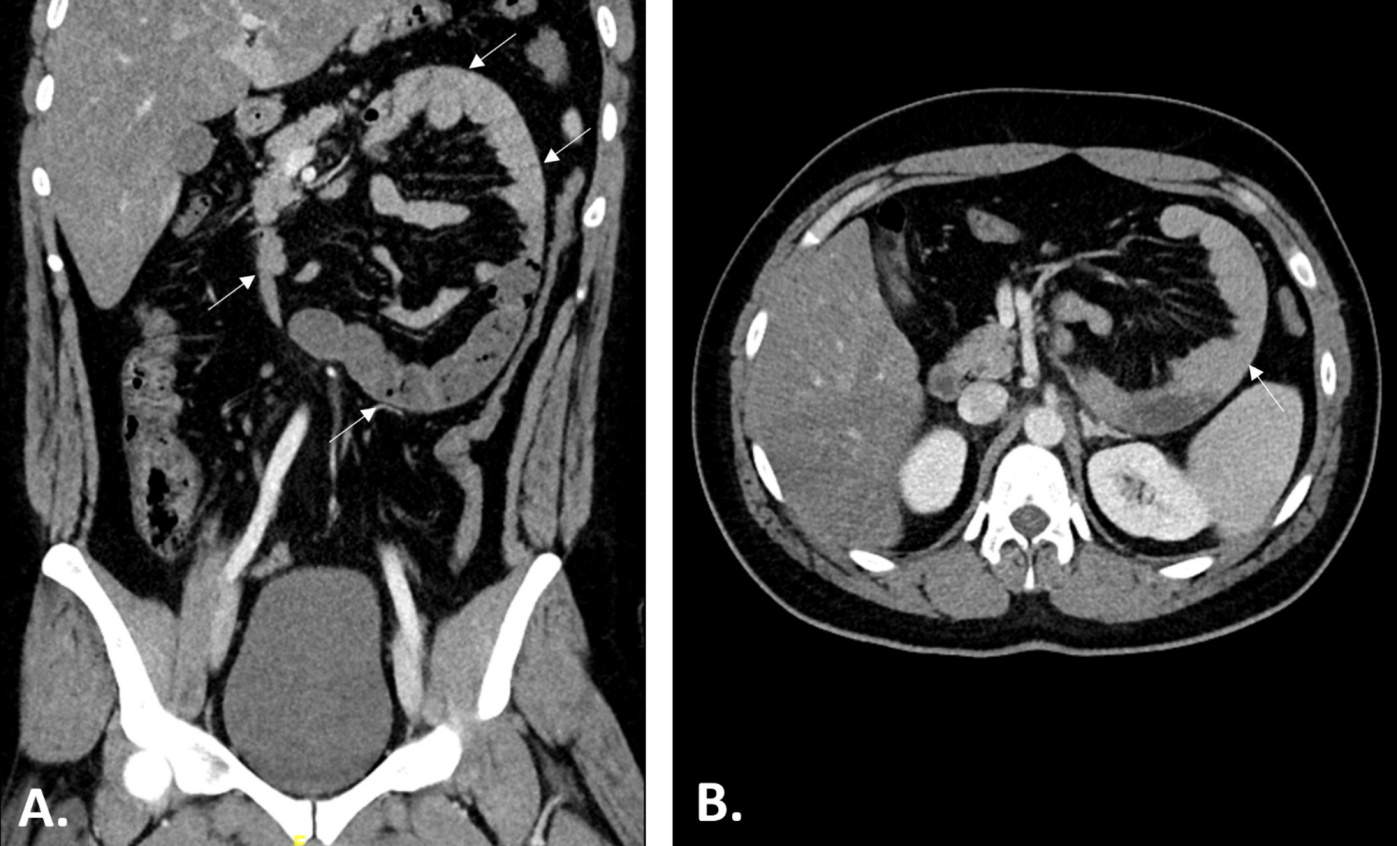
CT of the Abdomen (A: Coronal and B: Axial Images) CT images of the abdomen demonstrating sac-like clustered small bowel loops (arrows) noted in the left upper quadrant, in the anterior pararenal space. Also note that the remainder of large bowel is completely collapsed. These findings are suggestive of the diagnosis of left paraduodenal hernia.
